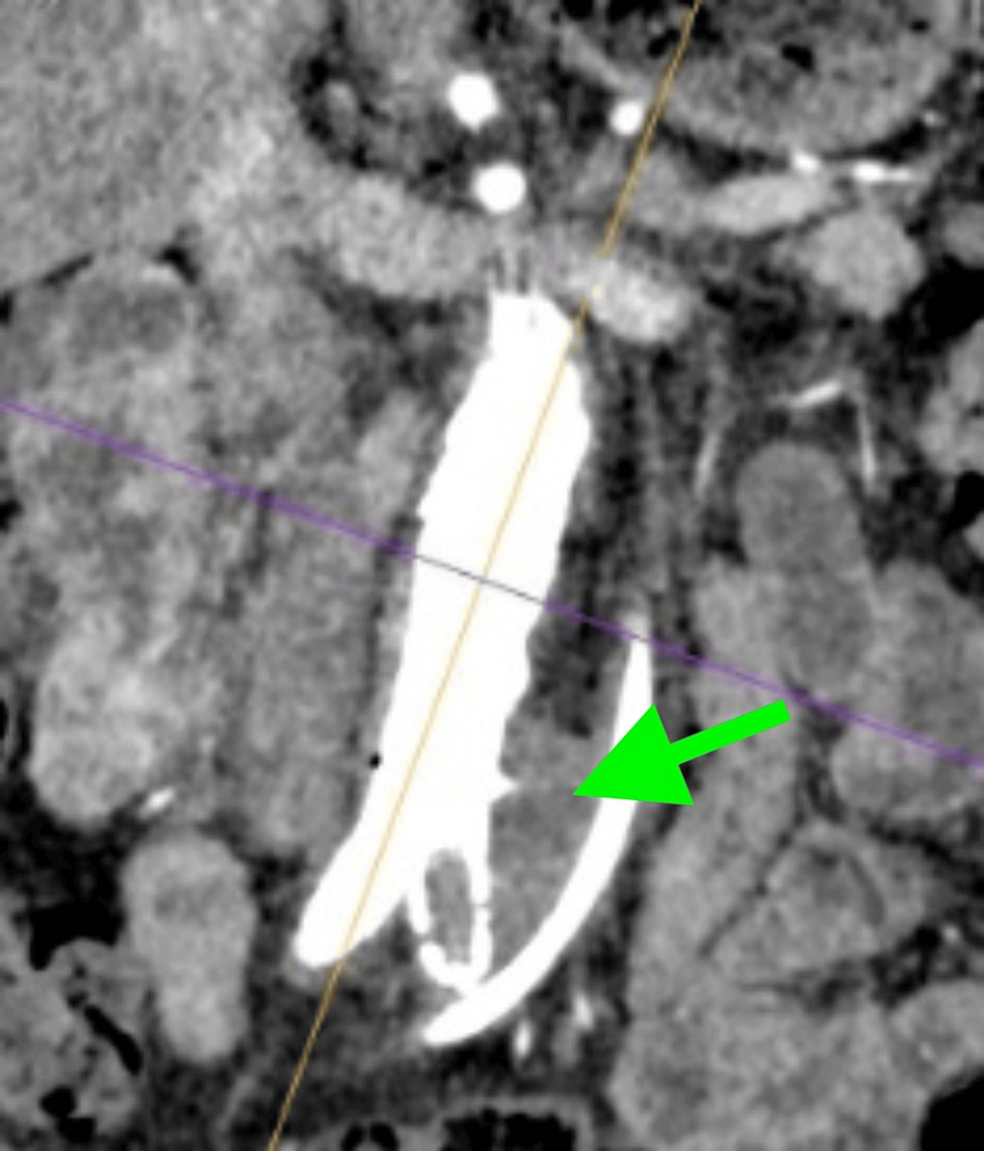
Post-procedural CTA showing control of the endoleak and no signs of extravasation in the area of AUF (green arrow). CTA: computed tomography angiography, AUF: aorto-ureteric fistula
